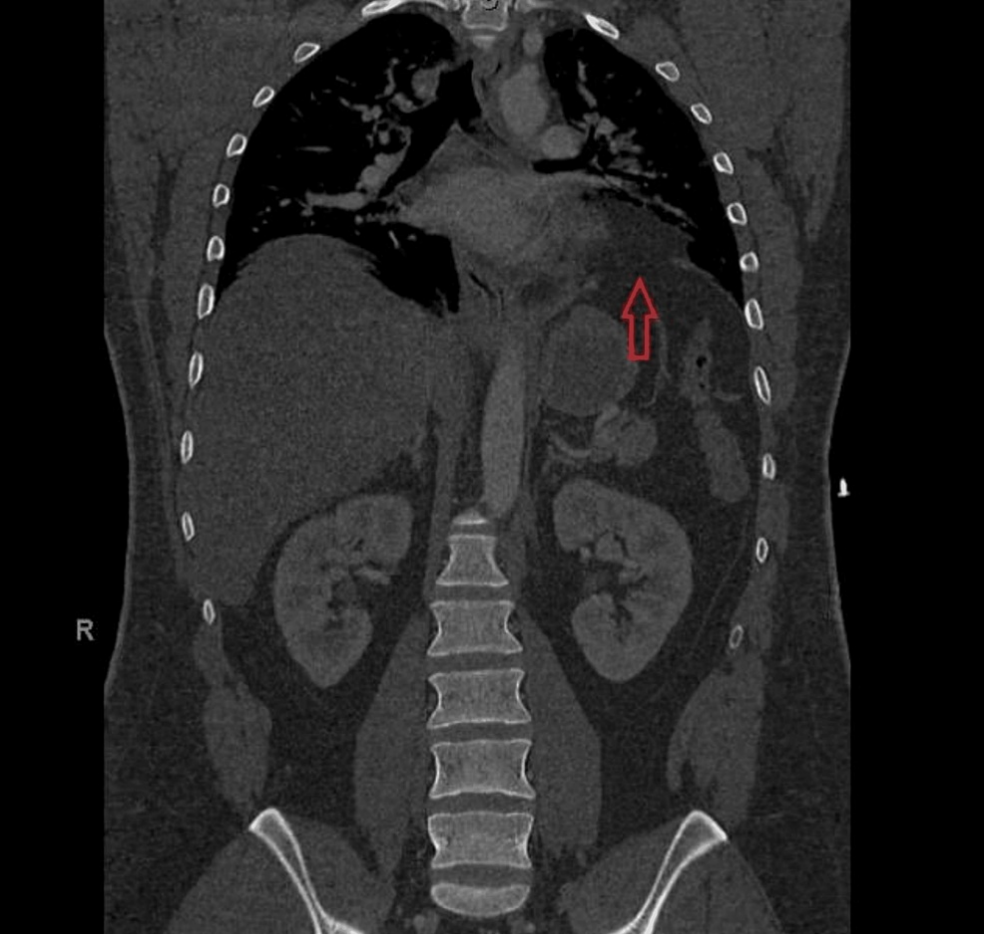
Coronal slice of CT scan on presentation, revealing left diaphragmatic discontinuity and a missed left traumatic diaphragmatic hernia CT - computed tomography
